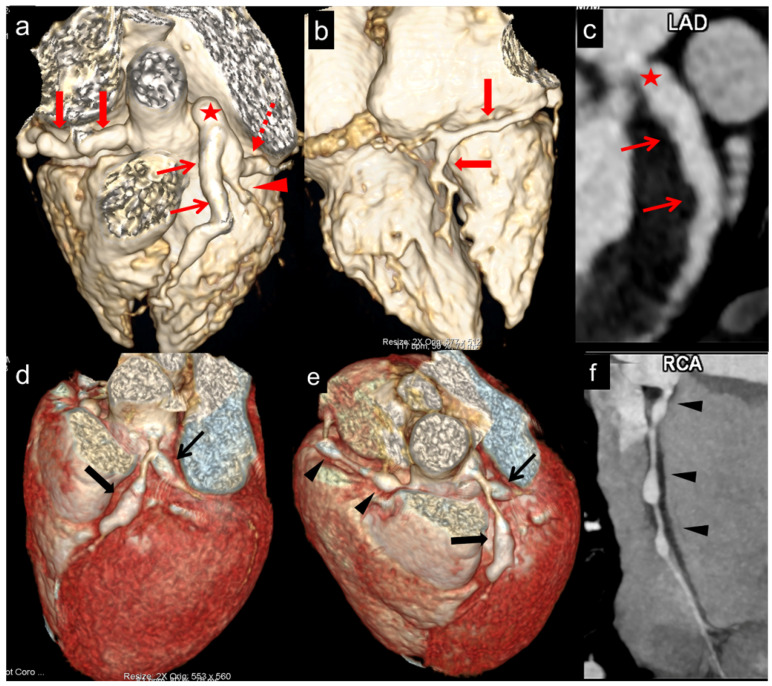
(a–c) (Patient No.3 in Table 2): CT coronary angiography (CTCA) volume rendered (a,b) and curved reformatted of left anterior descending (LAD) (c) images in a 7 months male infant at presentation show fusiform aneurysm of right coronary artery (RCA) along its entire course with extension into posterior descending branch (PDA) (thick arrows in a,b). The left main coronary (LMCA) (asterisk in a,c), proximal and mid anterior descending (LAD) (thin arrows in a,c), and proximal left circumflex (LCX) (interrupted arrows in a) show fusiform aneurysms. Fusiform aneurysm is extending to involve the proximal segment of the obtuse marginal (OM) branch of LCX (arrowhead in a). Although TTE showed aneurysms in LMCA, proximal LAD, LCX, and RCA, it failed to demonstrate the distal extension of the aneurysm of RCA and involvement of the OM branch of LCX. (d–f) (Patient No.15 in Table 2): CTCA volume rendered (a,b) and curved reformatted images of RCA (c) in a 76 months boy at presentation show fusiform aneurysms of proximal left anterior descending (LAD) (thick arrows in a,b) and left circumflex (LCX) (thin arrows in d,e). RCA shows three discontinuous fusiform aneurysms in the proximal and mid segments (arrowheads in e,f). Although TTE showed aneurysms in proximal LAD, LCX, and RCA, it failed to demonstrate aneurysms in mid RCA.
